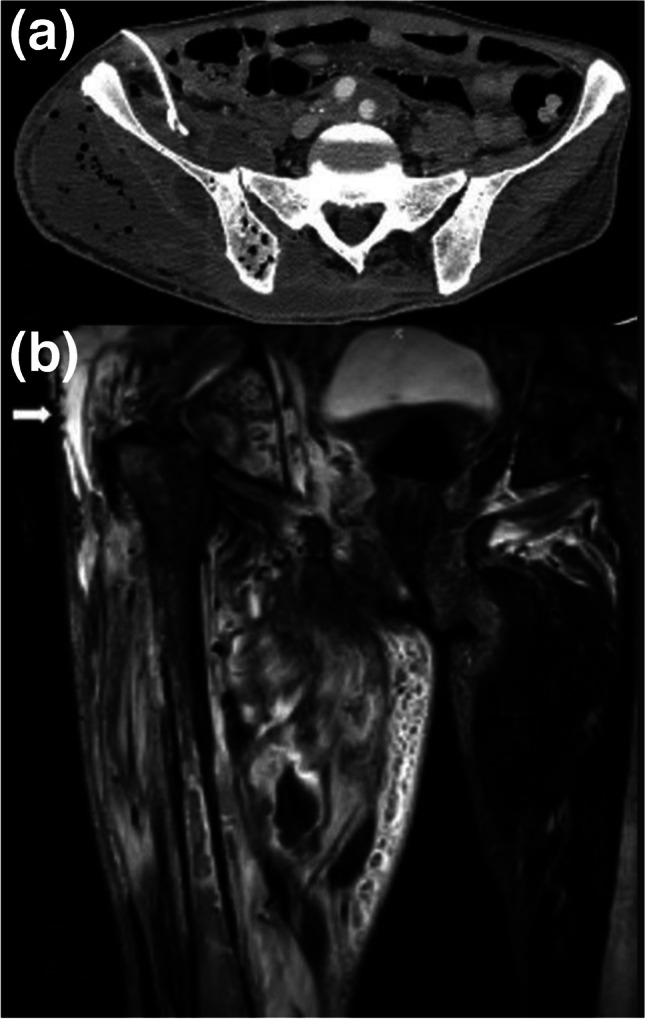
Axial CT (a) and coronal STIR (b) MR images of a 56-year-old man 3 months postangiography, performed via right femoral artery by Seldinger technique, are shown. Note the marked enlargement of the right gluteal and thigh muscles with low-density areas and air bubbles, compatible pyomyositis with abscesses and necrotizing fasciitis. The intraosseous air in the right iliac bone can be appreciated on the CT image as well as the drainage catheter in the right iliacus muscle abscess. Coronal STIR image (b) depicts that the extensive high-signal areas within superficial and deep soft tissue compartments, stranding within subcutaneous fat with a fluid collection in the right gluteal region (arrow), confirm pyomyositis, abscess, and necrotizing fasciitis and cellulitis. Multiple air sacs appear as signal-void areas within the medial thigh muscles on the MR image (b)
